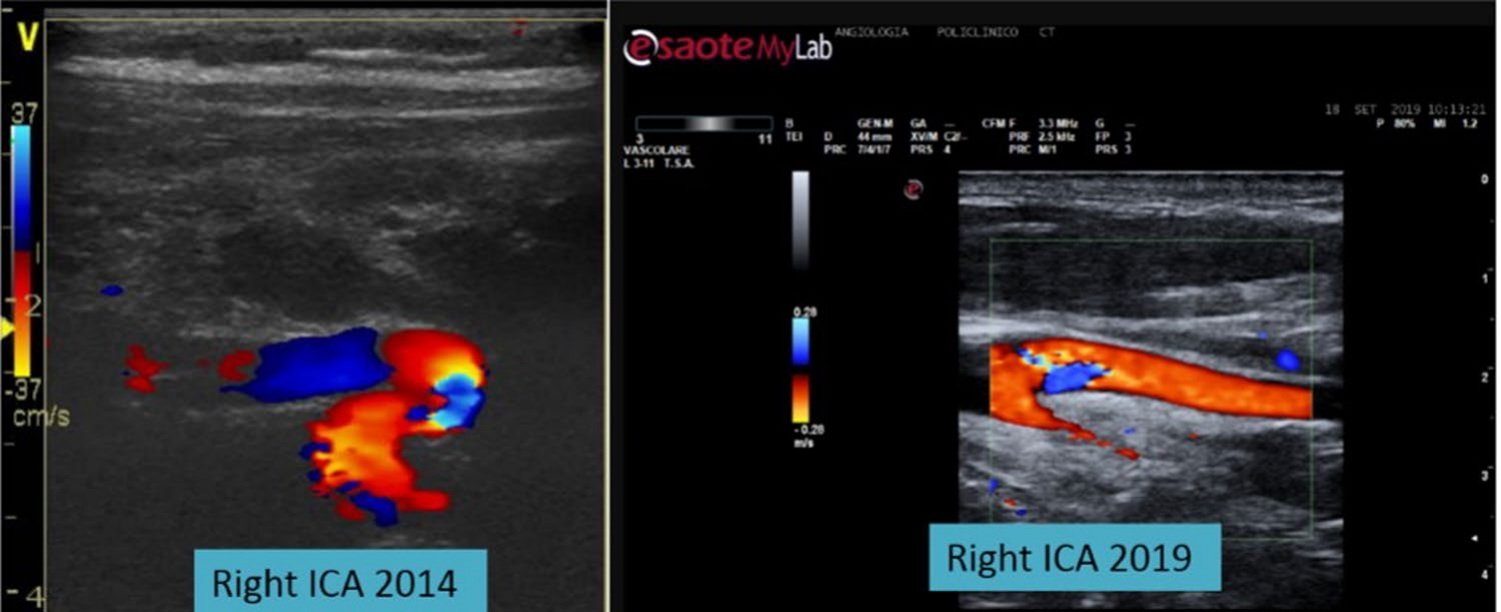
The disappearance of the internal carotid artery (ICA) kinking in a child five years after the first echo-color Doppler (ECD) study. This figure shows the image of ECD study of the right internal carotid artery (ICA) of a child in two different times in his life. a At the age of 11 years old (in 2014), he presented a morphological anomaly of right ICA with an angulation < 90°, i.e. a kinking. b Five years later (in 2019, when he was 16 years old), the ECD study of the same subject’s right ICA showed a total disappearance of the dolichoarteriopathy
